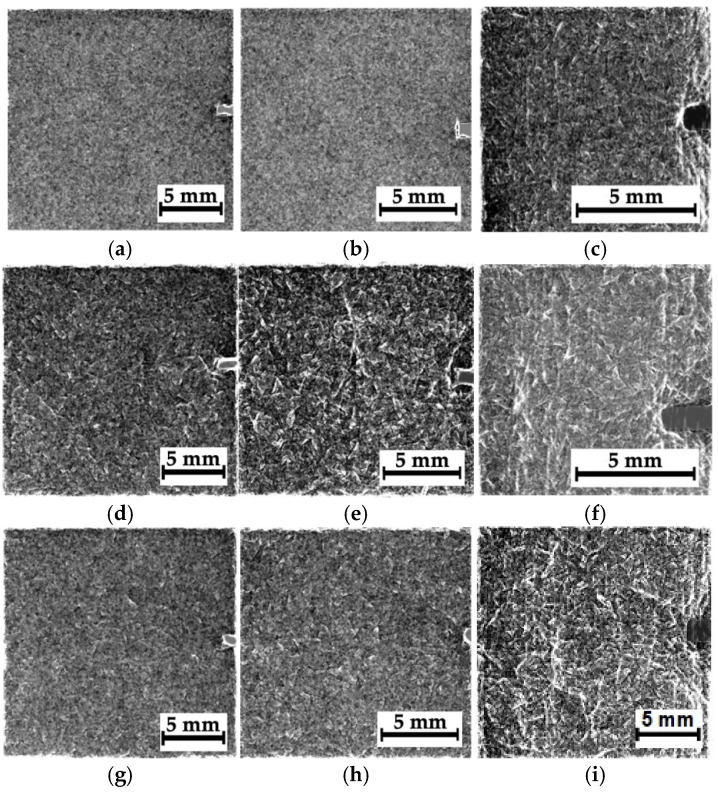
The phase variations of the processed radiographic images shown in Figure 8a–c; the distances between the sample and the sensor are 39 mm (a,d,g), 160 mm (b,e,h), and 240 mm (c,f,i).
